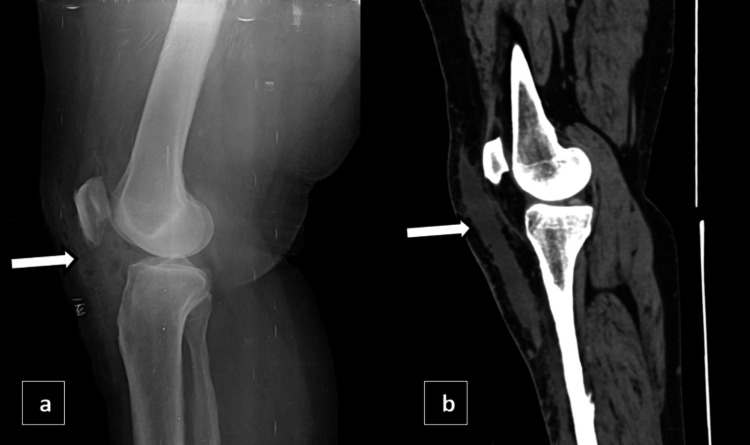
X-ray knee lateral view (a) and CT knee joint sagittal view (b) showing ill-defined haziness in the suprapatellar and infrapatellar regions with the corresponding CT showing a fairly defined hypodense fluid collection between the subcutaneous and intermuscular planes represented by white arrows.
